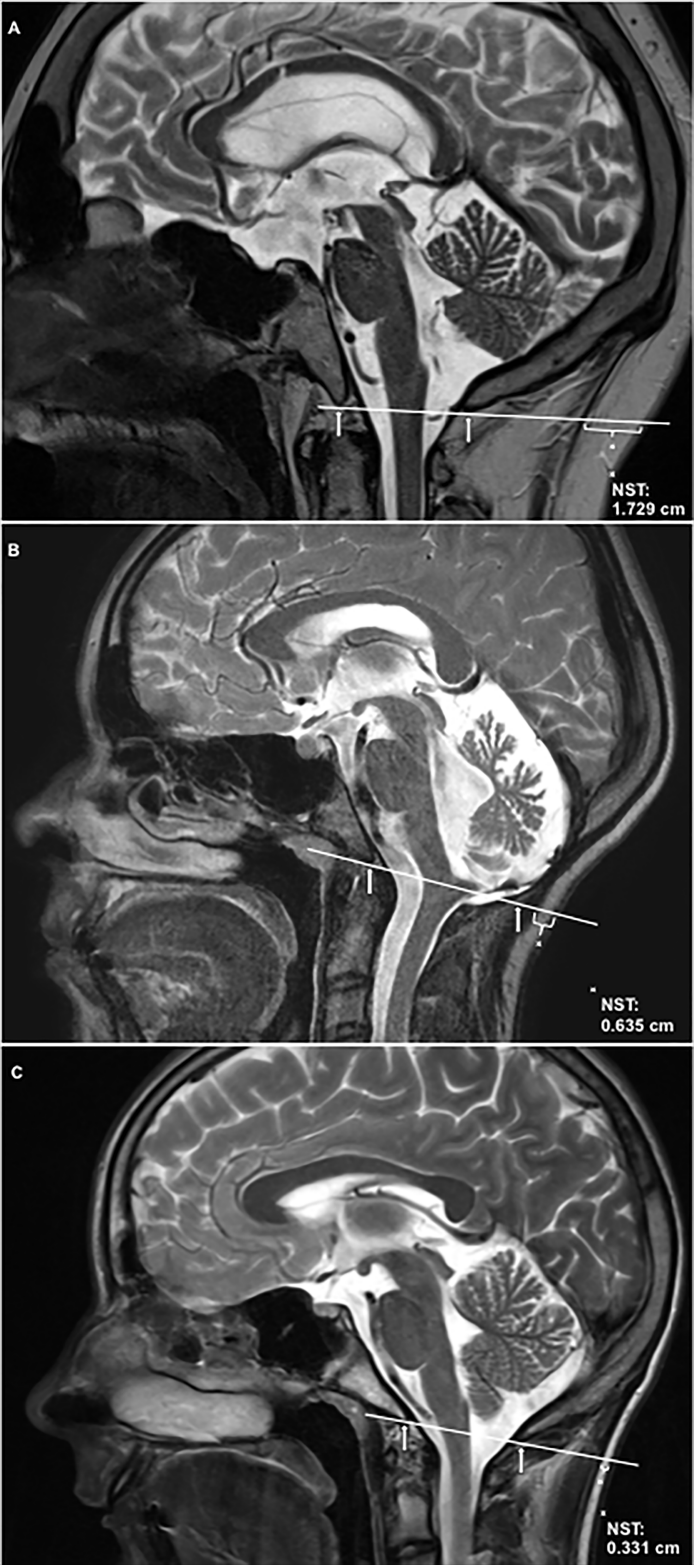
Nuchal skinfold thickness (NST) is shown on T2-weighted sagittal cranial magnetic resonance imaging (MRI) of the midline. NST was quantified according to the following standardized assessment: First a line was drawn crossing the two anatomically defined points: basion (anterior margin of the foramen magnum, indicated by arrow) and opisthion (posterior margin of the foramen magnum, indicated by arrow). The diameter of subcutaneous nuchal fat was measured over this line to the nearest 0.01 cm using OsiriX (Pixmeo SARL, Switzerland). (A) shows NST in a childhood-onset craniopharyngioma patient with severe obesity due to hypothalamic involvement [hypothalamic involvement grade II (13), body mass index (BMI): +4.86 SDS (32)]. (B) shows NST in a patient with low-grade glioma of the brain stem, BMI: +0.48 SDS. (C) shows NST in a healthy, normal-weight control [BMI: +0.41 SDS (32)]. SDS, standard deviation score. The inter-rater reliability of the used arithmetic mean of NST was 0.982.
